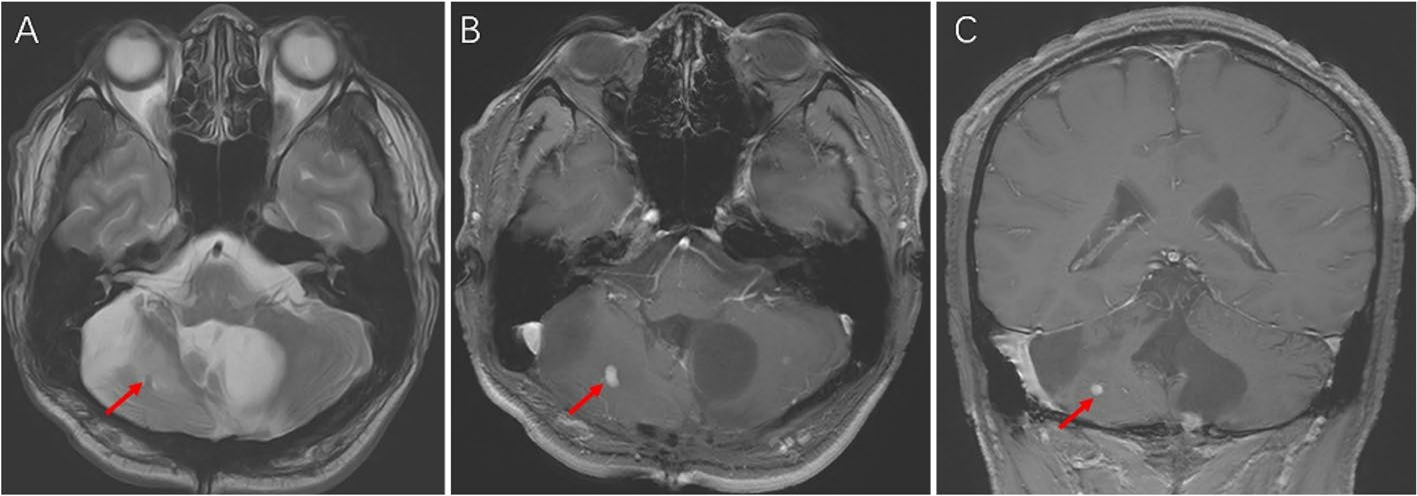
Cranial MRI. A T1W1. Low signal occupation in the right cerebellar hemisphere (arrow). B T2W1. High signal occupation in the right cerebellar hemisphere (arrow). C Sagittal position. Large sacs and small nodules, and mural nodules were obviously enhanced
